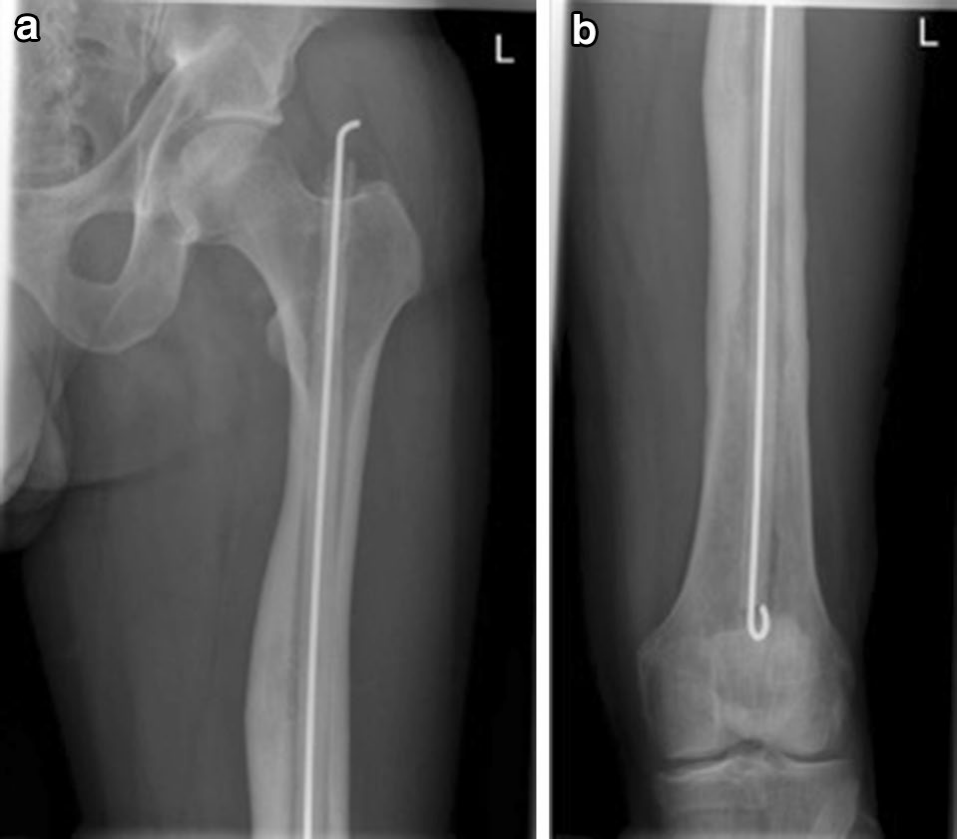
a, b Immediate postoperative radiographs of the *left* femur showing the rod with antibiotic impregnated cement that was used for local antibiotic delivery
